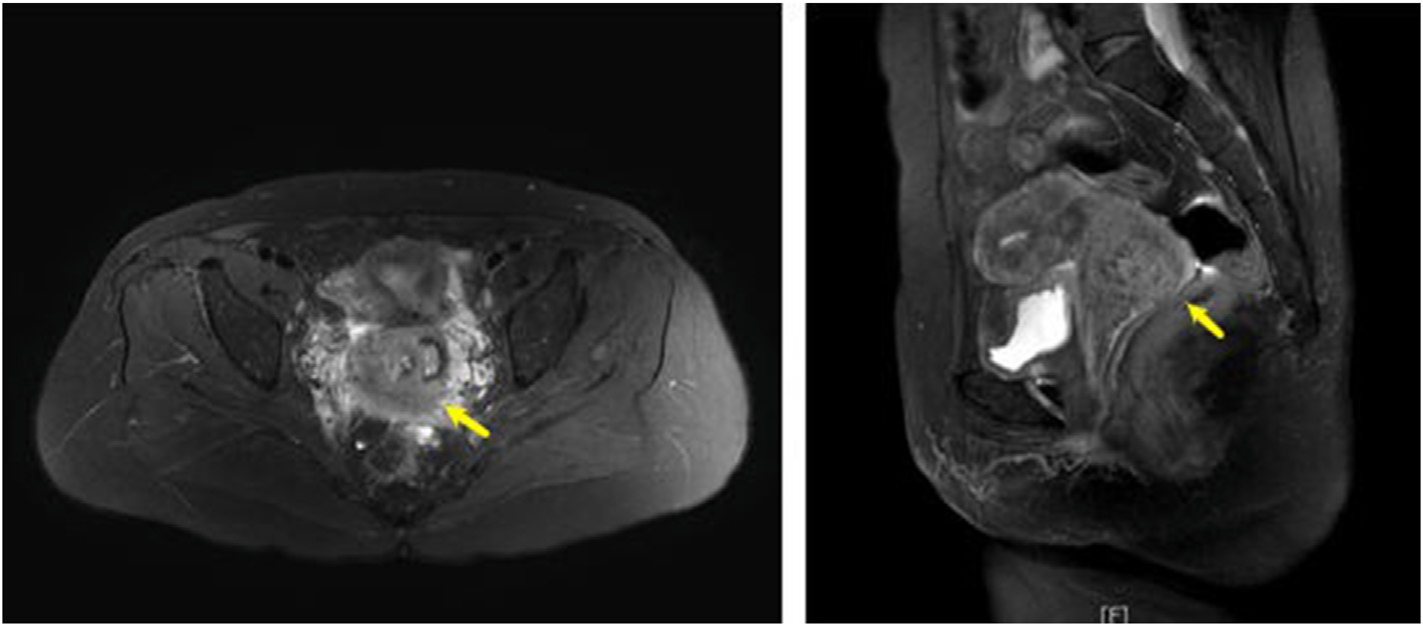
The pelvic 3T MRI showed a 3.7 × 3.6 × 4.0 cm necrotic mass with internal heterogeneous signals on T2-weighted image. (a) Axial view (b) Sagittal view.
